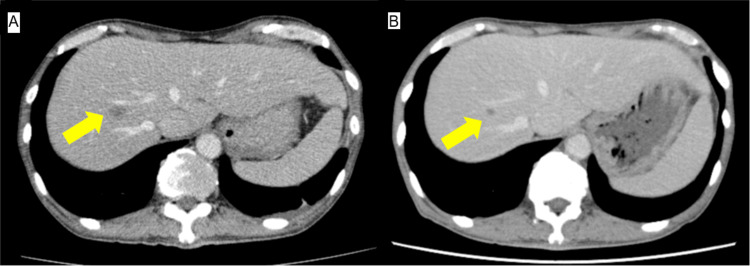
Abdominal computed tomography (A, B) Abdominal computed tomography reveals shrinkage of the known liver metastasis (arrow), and no new metastasis was found.
